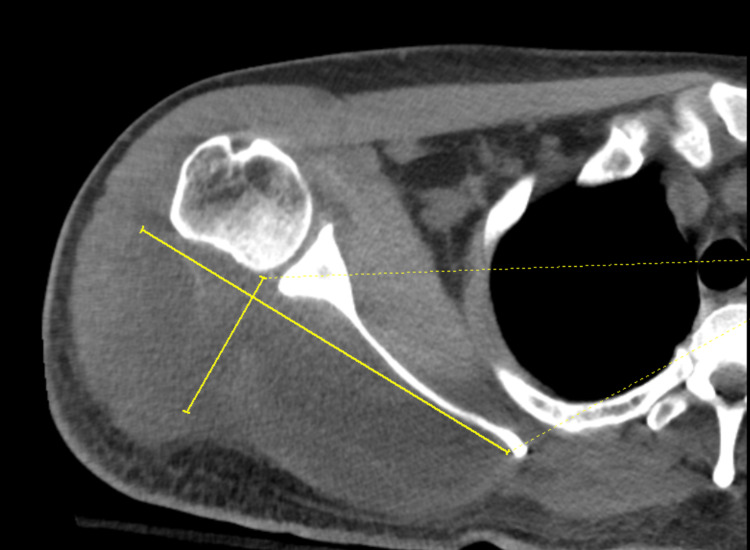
CT of the right shoulder showing a hypodense intramuscular collection (yellow lines marking length and breadth of the collection) with no signs of gas, concerning for intramuscular hematoma versus infection specifically in the infraspinatus muscle.
